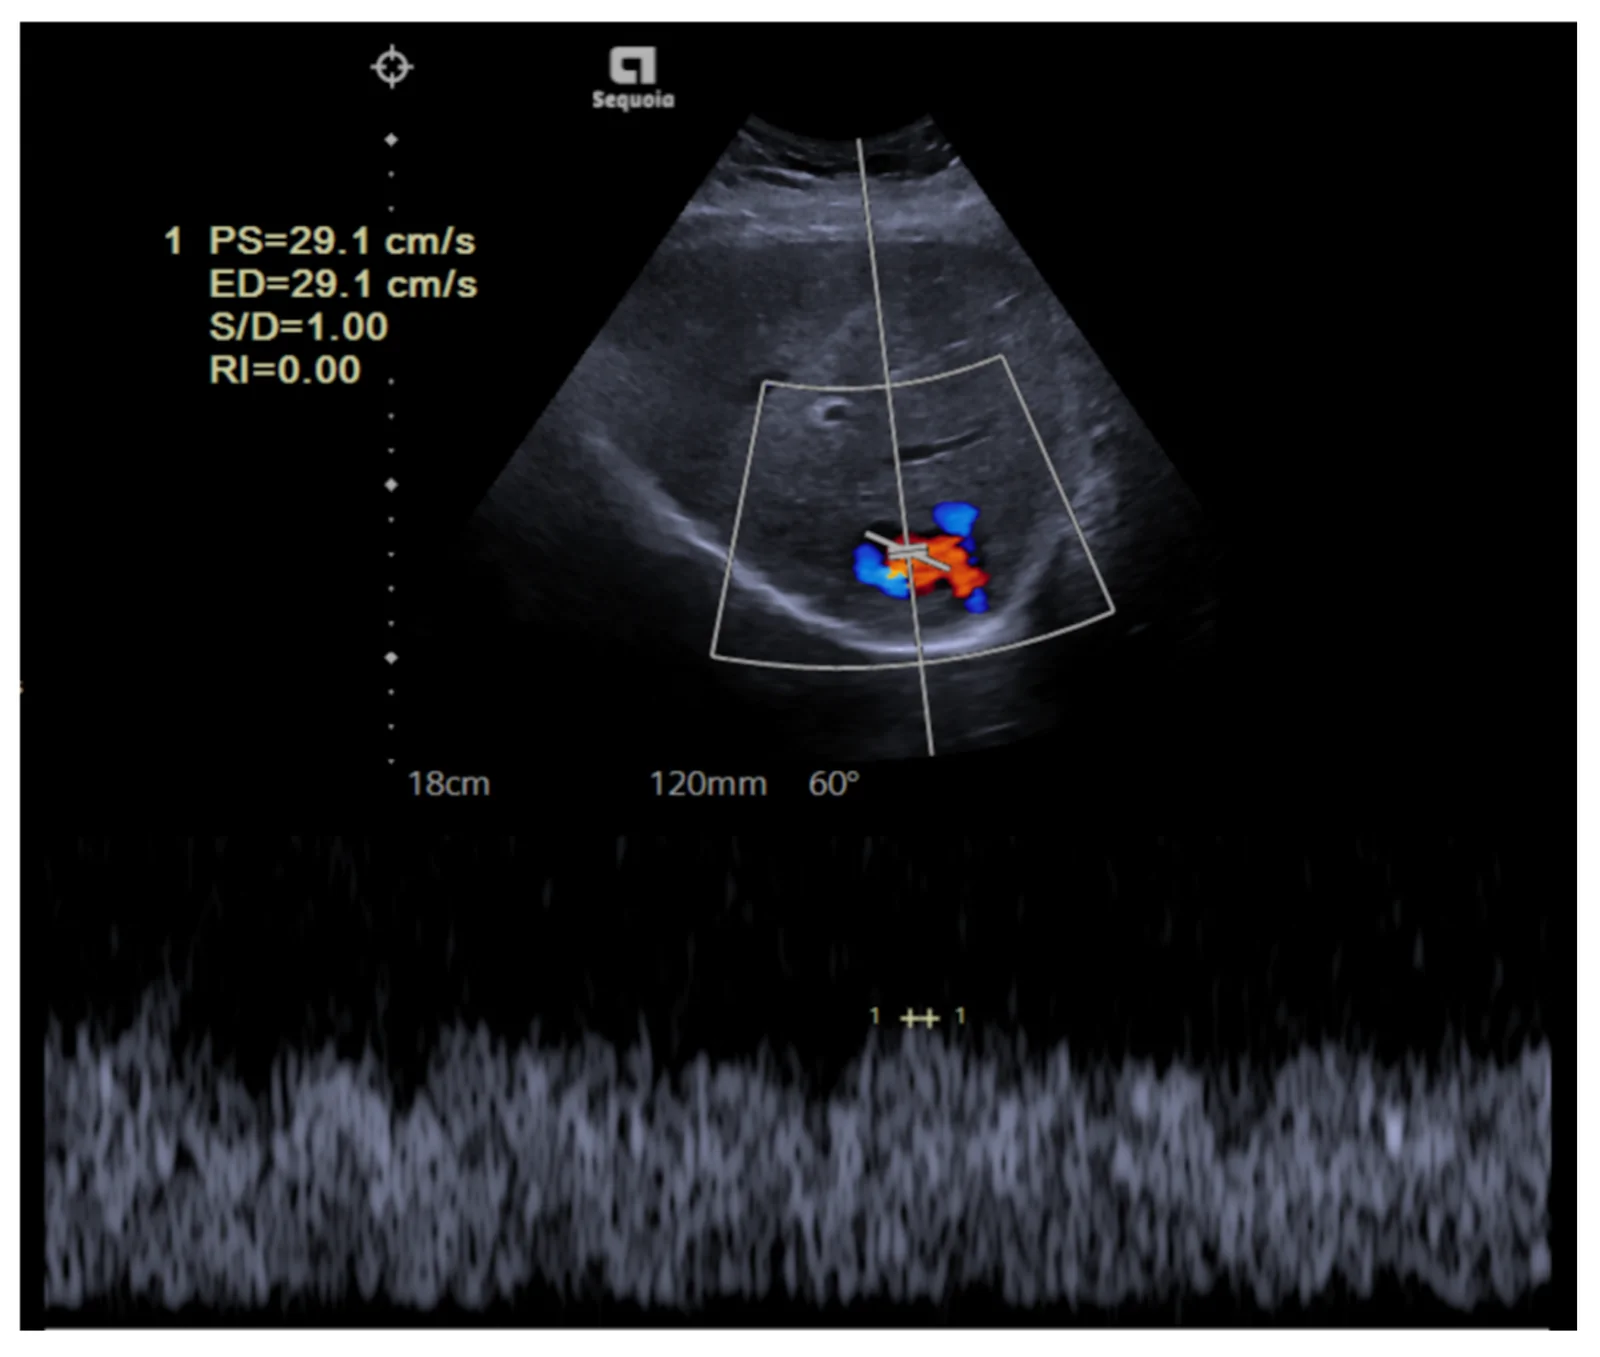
Color Doppler ultrasound of the cystic lesion showing an apparent connection with the hepatic venous system.
